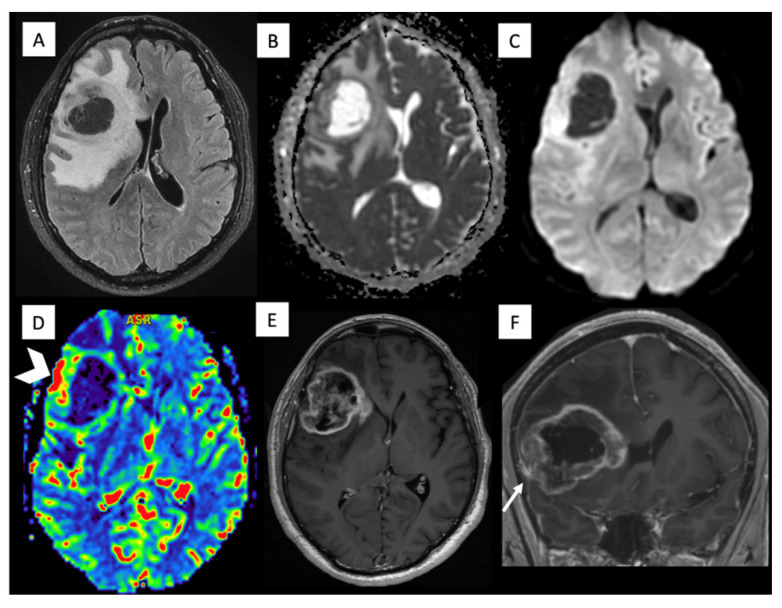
Brain MRI of Patient 1. Brain MRI axial fluid-attenuated inversion recovery (FLAIR) (A), ADC (B), diffusion b1000 (C), DSC perfusion (D) and axial and coronal contrast-enhanced T1-weighted (E,F) images show a right frontal lobe mass with a necrotic center and peripheral enhancement, edema, restricted diffusion, increased rCBV (arrowhead), and meningeal extension (arrow).
